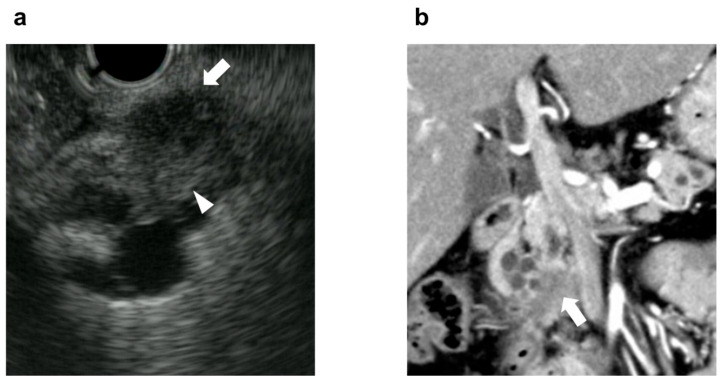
In this study, an irregular mass in the pancreatic parenchyma that was contiguous with a mural nodule was considered an SC. (a) EUS showing an SC (arrow) in the pancreatic parenchyma that is contiguous with the mural nodule (arrowhead). (b) CT showing an SC (arrow) infiltrating the pancreatic parenchyma from within the cyst. SC, solid component; EUS, endoscopic ultrasonography; CT, computed tomography.
